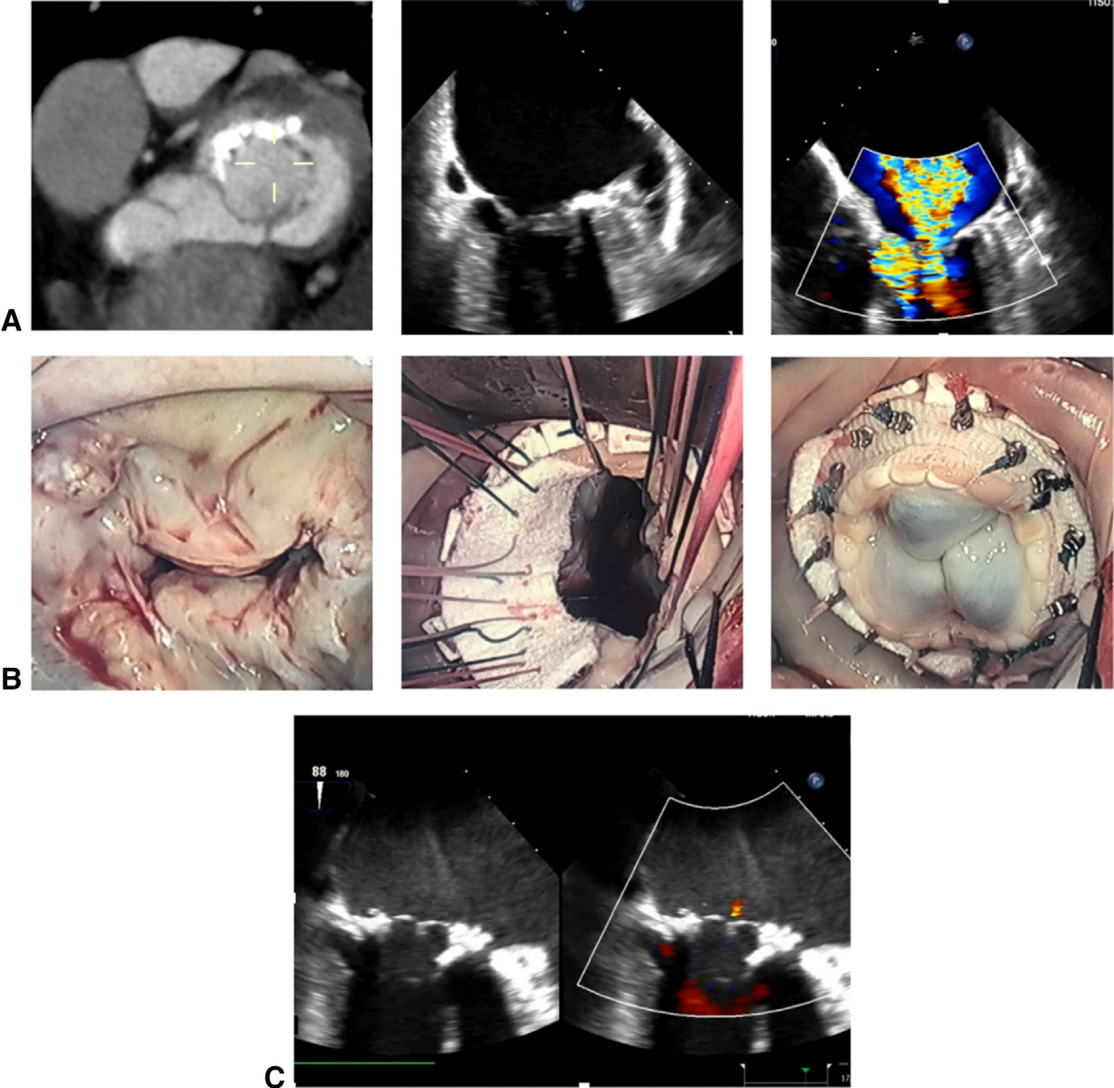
Use of ultrasonic emulsification. A, Preoperative CT and 2D echocardiogram demonstrating focal MAC (*right* and *middle panel*) with MR (*left panel*). B, Intraoperative images demonstrating initial MAC (*right panel*), followed by placement of a 29-mm Epic valve via a minimally invasive approach (*middle* and *left panel*). C, Postoperative 2D echocardiogram demonstrating resolution of MR.
